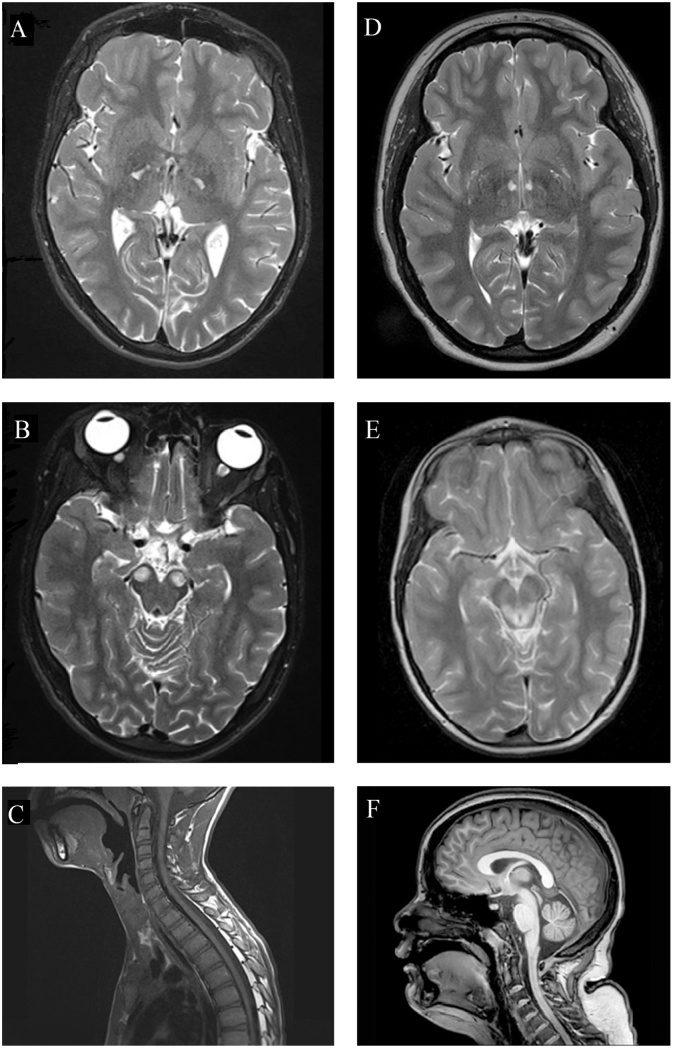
MRI studies. Brain MRI studies of proband 1 (A–C) and proband 2 (D–F). Brain MRI of proband 1 at 19 years of age showing diffuse brain atrophy (A) and T2-hyperintense lesions in the anterior medial part of the mesencephalon (B). MRI of the spinal cord of proband 1 at 14 years (C). Brain MRI of proband 2 at 15 years of age showing small lesions bilaterally in the inferior thalamic region (D). Brain MRI at 6 years with T2-hyperintense lesions of the tegmentum and the periaqueductal grey matter (E), and mild cerebellar atrophy (F).
